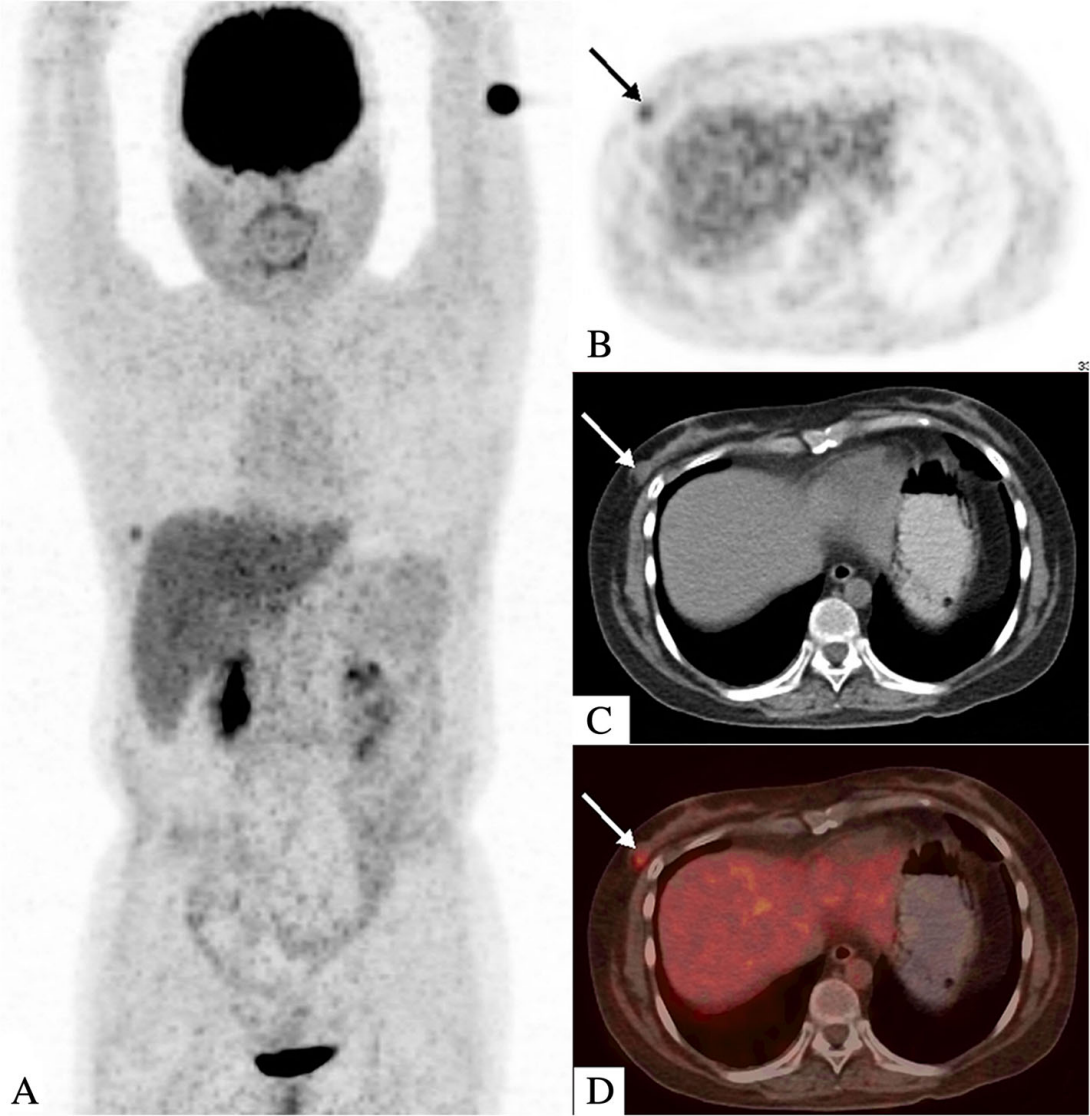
Whole-body positron-emission tomography/computed tomography (PET/CT) findings. a The PET/CT scan demonstrated a hypermetabolic nodule in right breast and no focal uptake in other sites. The 18 F-FDG-avid nodule was located in the outer upper quadrant of the right breast with a SUV of 4.6 (b, PET images; c, CT images; d, fused PET/CT images)
